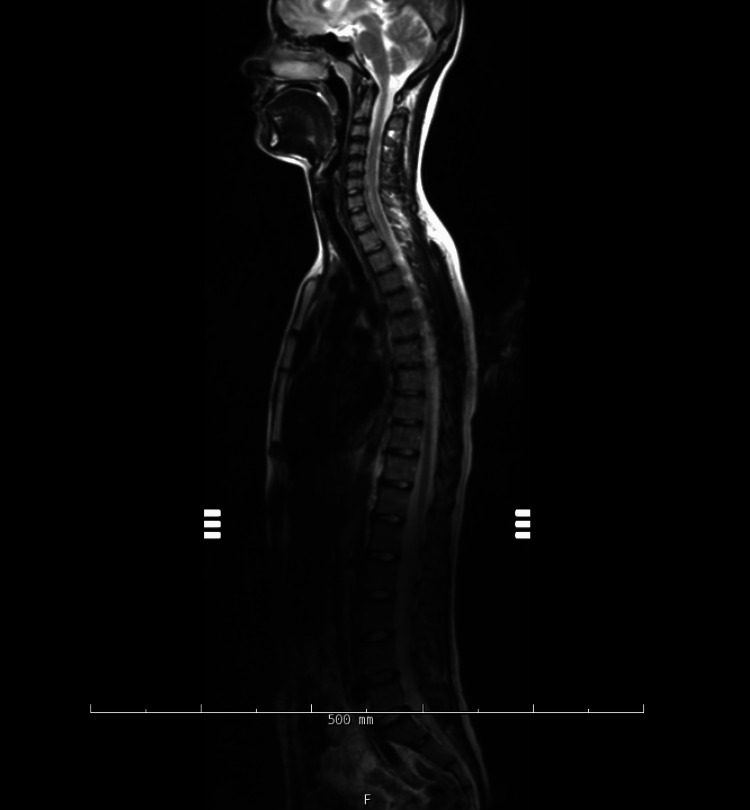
T2 MRI entire spinal cord without IV contrast
